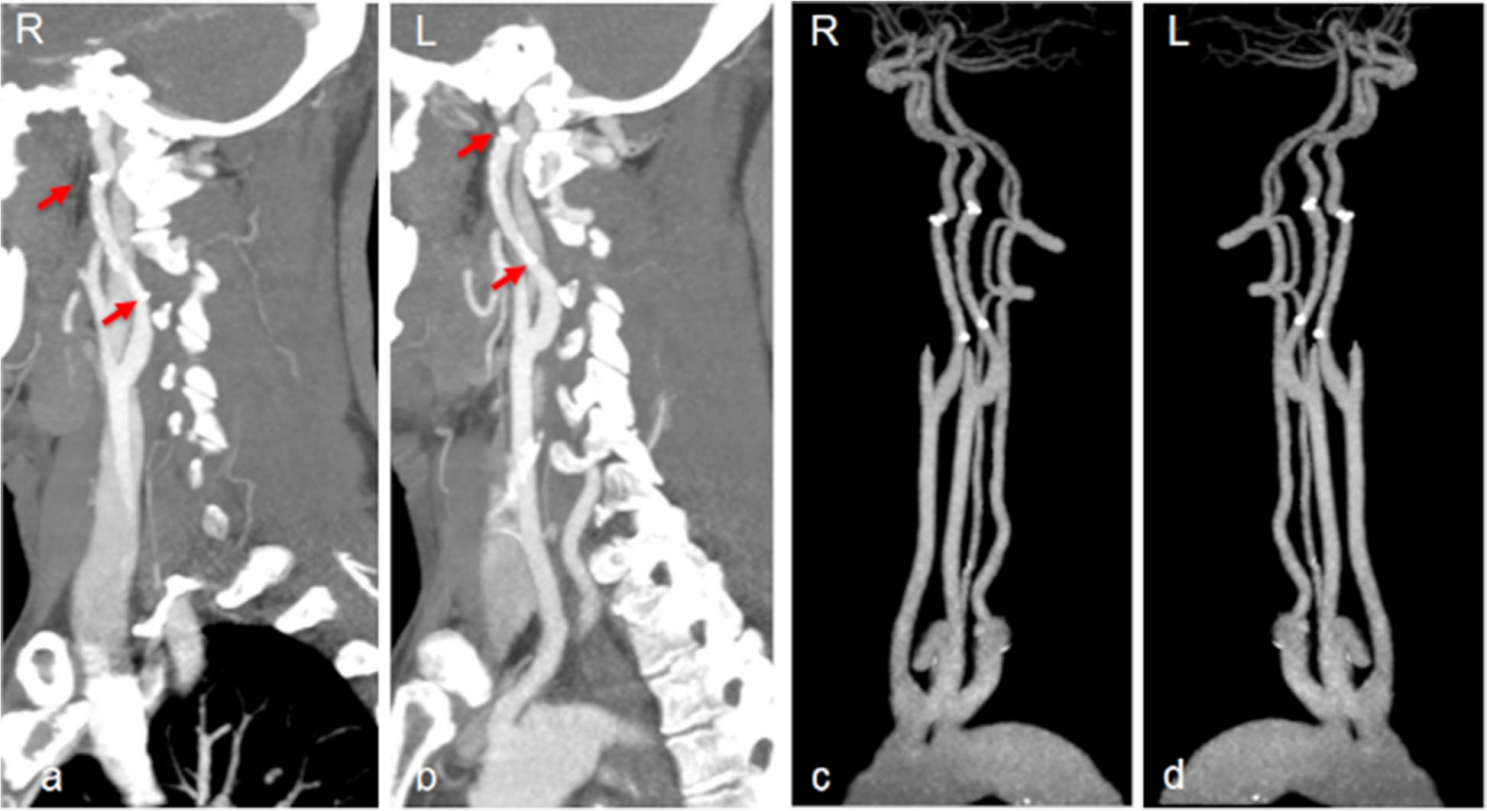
Follow-up images. a-b Follow-up CTA at three months showed that bilateral ICAs were repaired well (Nov 26, 2020); c-d Follow-up CTA at 15 months showed that bilateral ICAs remained patent and no dissection relapsed (Dec 13, 2021)
